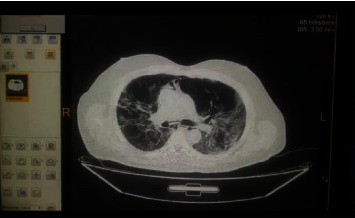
Lung CT scan for a patient with confirmed COVID-19 infection.
